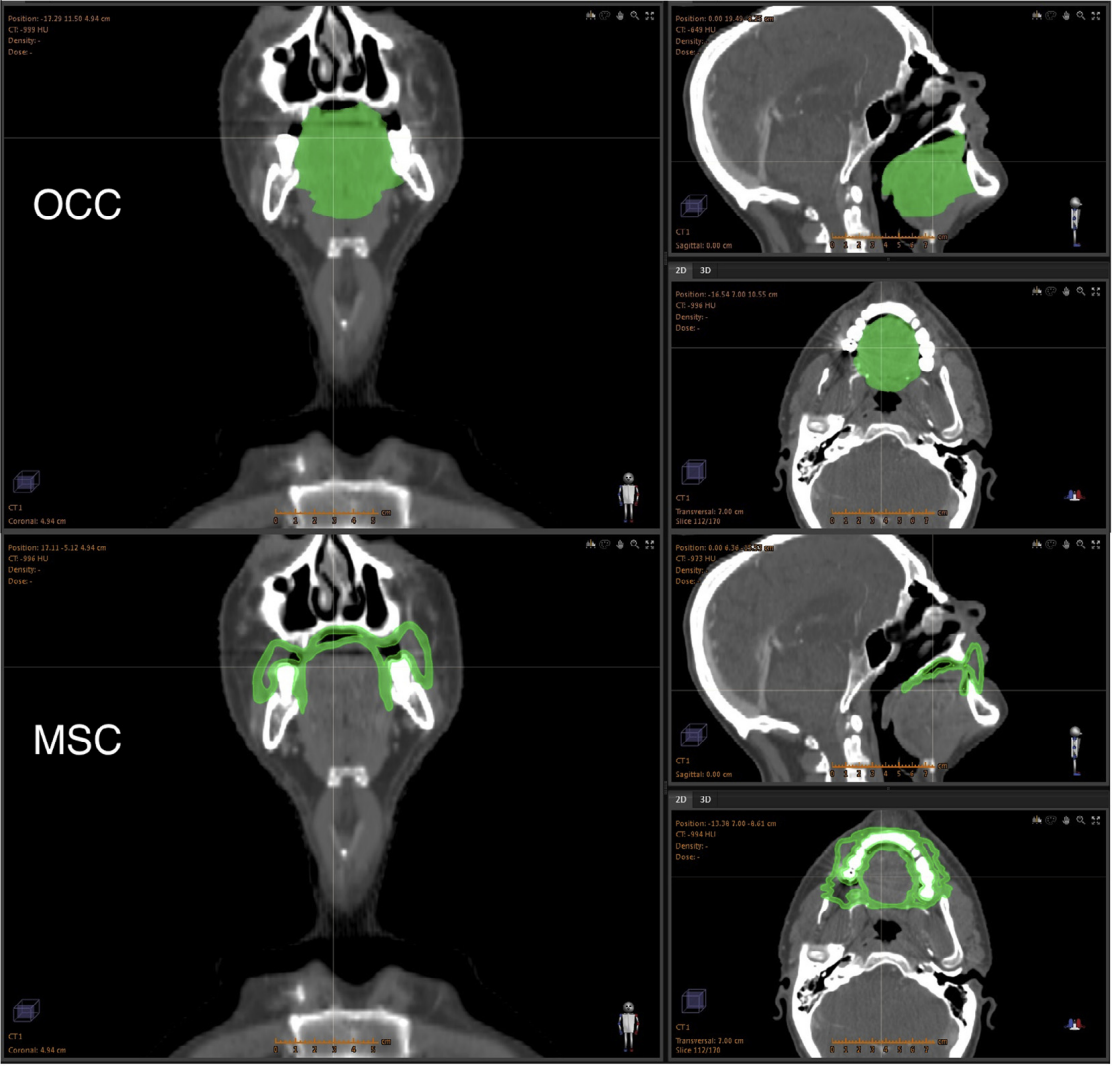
CT scan of a head and neck radiotherapy patient with the OCC (top) and MSC (bottom) shown in green. The OCC representation reduces the complex shape of the mucosal wall to a simplified solid volume, whereas the MSC structure is represented as a mucosal surface and, as such, represents a more anatomically realistic description of the oral mucosa.
